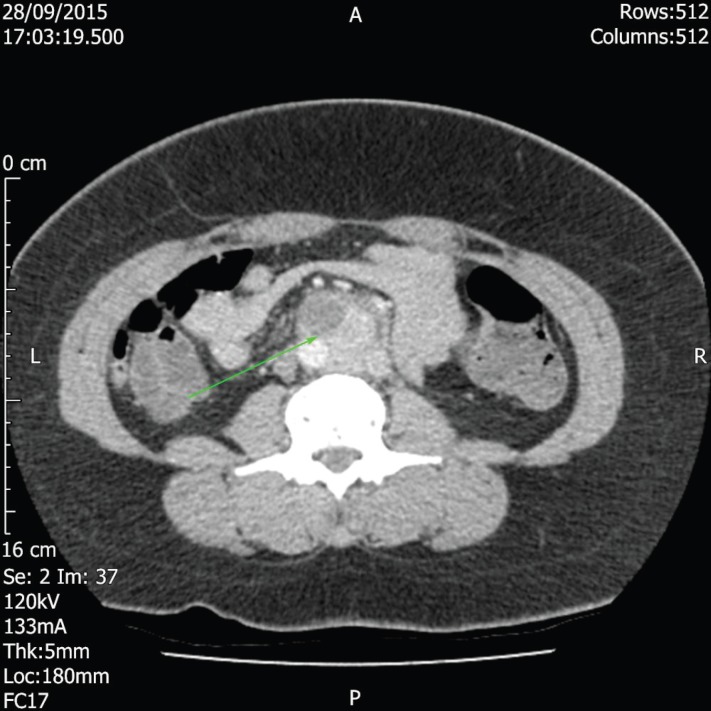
CT Image demonstrating retroperitoneal mass
